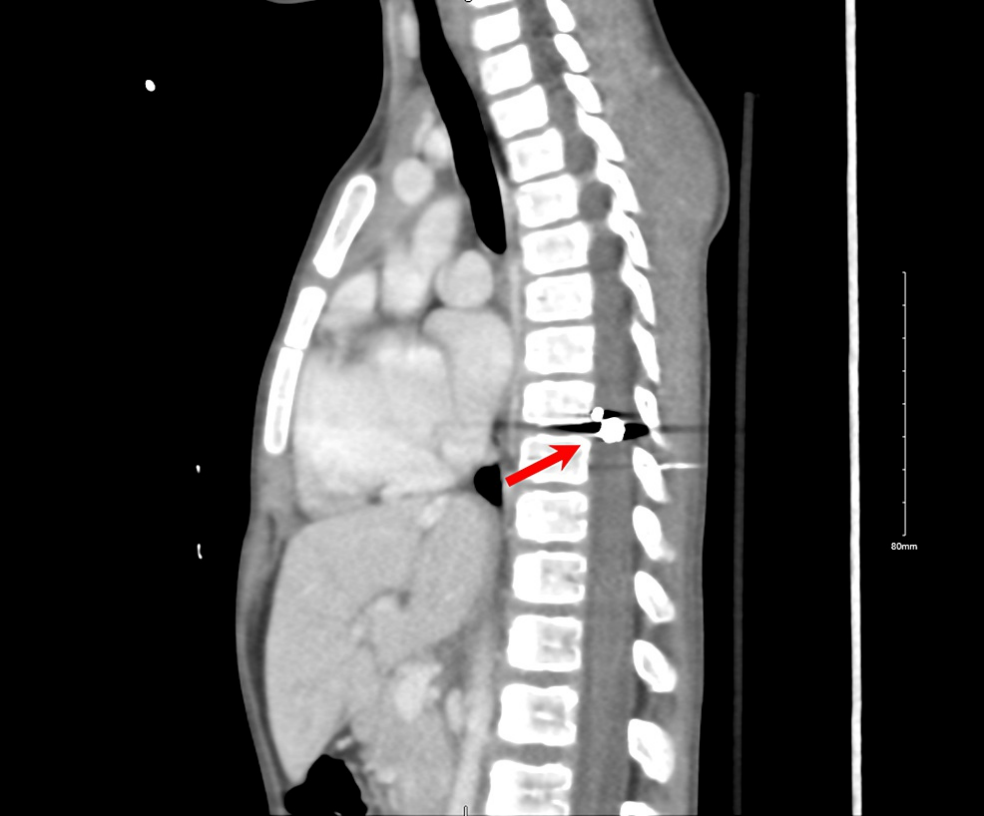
Sagittal CT scan showing the fragmented bullet at the level of T8.
